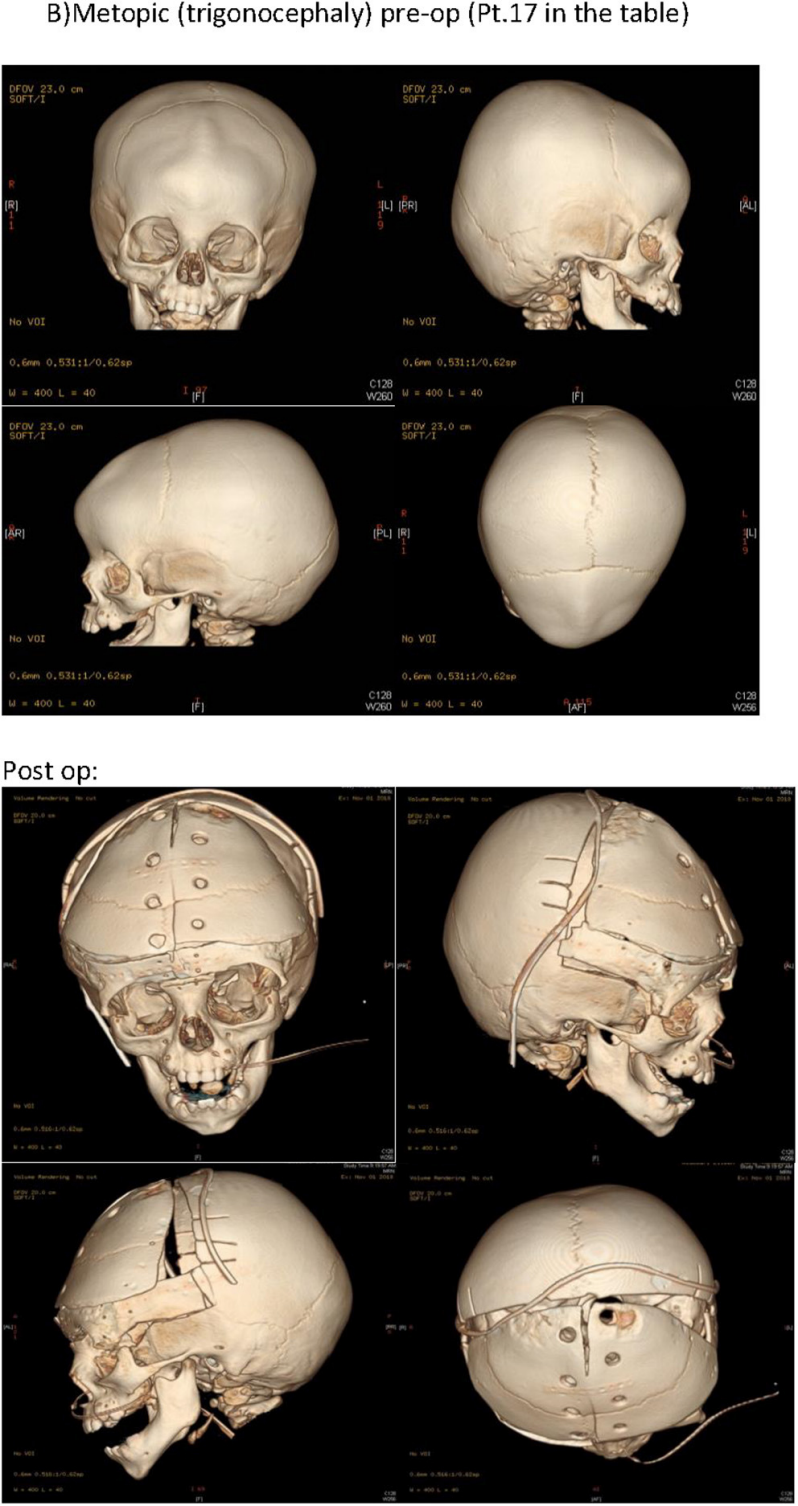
Pre-operative CT scan was done for all patients, along with 3D printed models to aid in patient explanation and decision making. Aperts: pre-op and post-op. CT (Pt.4 in the Table 1). B) Metopic (trigonocephaly) pre-op and post-op CT (Pt.17 in the Table 1).
